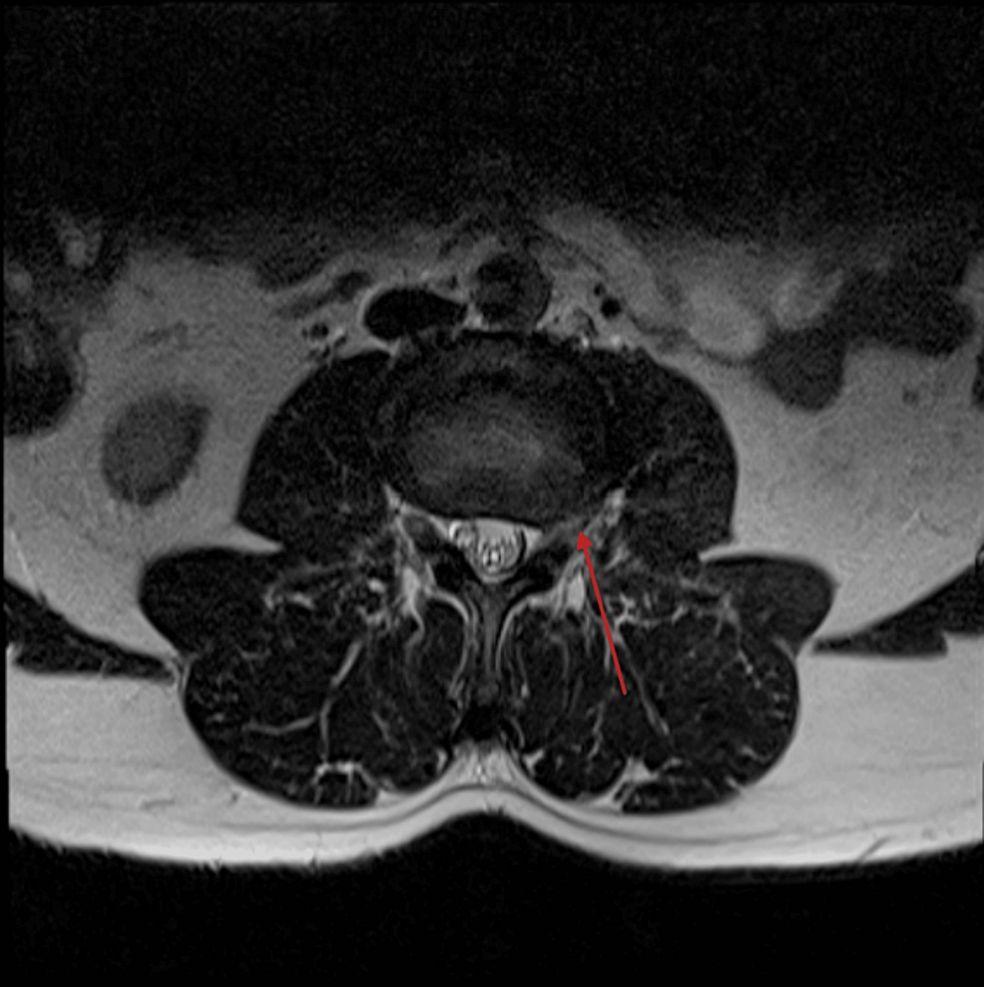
MRI spine axial section T2 sequence showing small (<1 cm) left far foraminal disc at L3/L4, which is impinging on the exiting L3 nerve just after it enters the exit foramen.  Image source: Case courtesy of Ian Bickle, Radiopaedia.org. From the case https://radiopaedia.org/cases/44881?lang=gb rID: 44881 MRI: Magnetic resonance imaging
